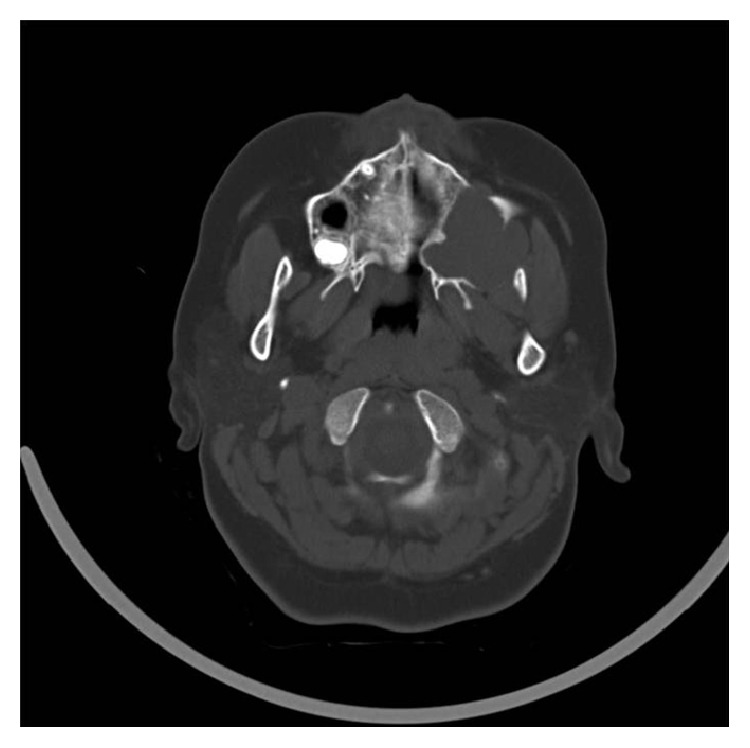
Axial section of CT scan showing osteolytic changes.
